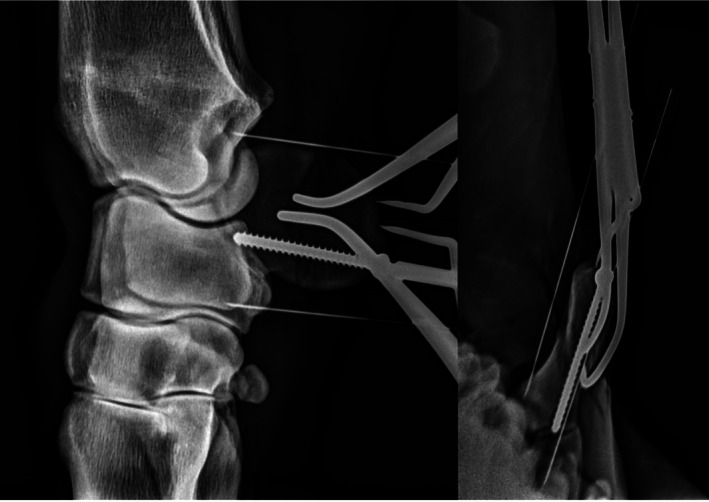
Intraoperative radiographs of repairing a vertical plane fracture of the accessory carpal bone (ACB). Left image: Radiograph in latero‐medial projection. The insertion of a cortical screw in lag fashion from palmar to dorsal into the distal portion of the ACB was guided by spinal needles which were placed proximally and distally to the ACB for drill orientation. Right image: Radiograph in D80°Pr30°L PaDiMO. Two spinal needles, one proximal and one distal to the ACB, were used to orientate the cortical screw, which was inserted between the lateral and medial cortex of the ACB in a palmar‐to‐dorsal direction.
